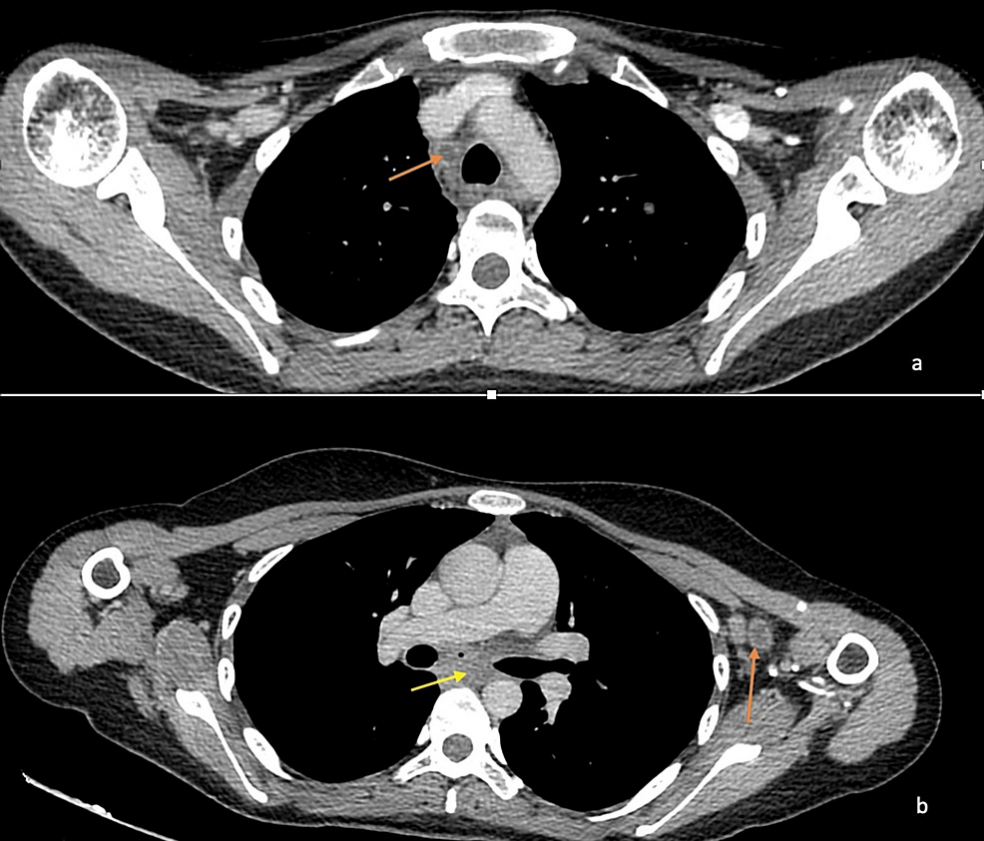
Mediastinal and left axillary lymph nodes due to tuberculosis on the axial sections of the CT chest Axial sections of the CT chest showing (a) a right paratracheal lymph node (orange arrow). A note is made of the central hypodensity within the lymph node suggestive of necrosis and the faint rim of contrast enhancement. (b) A large necrotic lymph node in the left axilla (orange arrow). A subcarinal lymph node is noted as well (yellow arrow).
